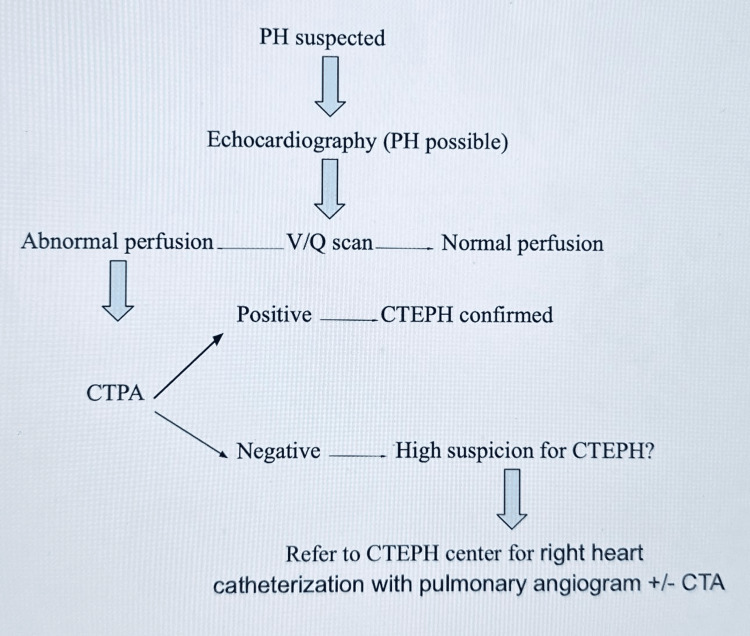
Algorithm of CTEPH diagnosis PH: pulmonary hypertension, V/Q: ventilation/perfusion, CTPA: computed tomographic pulmonary angiography, CTEPH: chronic thromboembolic pulmonary hypertension, CTA: computed tomography angiography.
